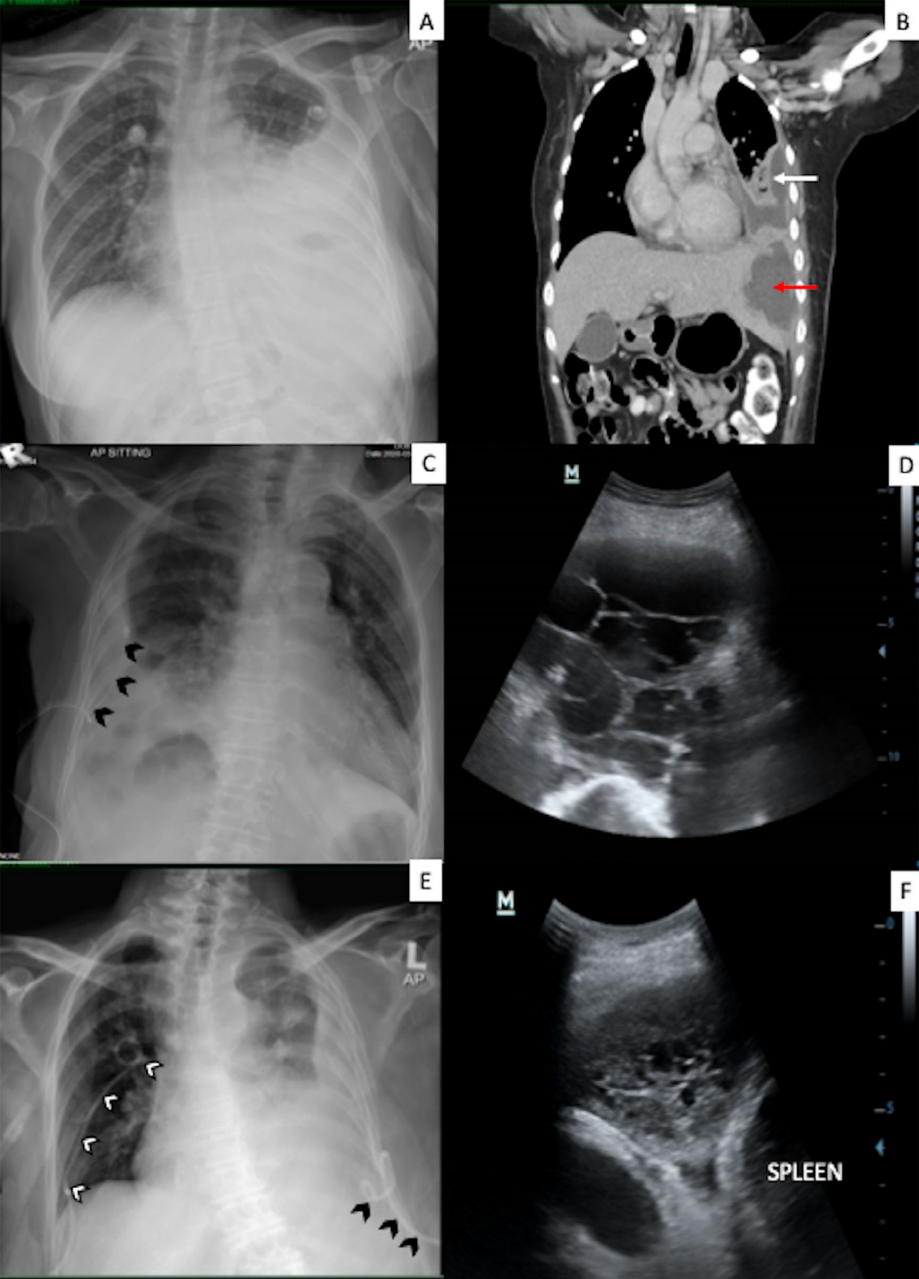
Imaging before intrapleural fibrinolysis. Patient 1: Chest radiograph showed massive left pleural effusion (A) and computed tomography (CT) (B) showed loculated left effusion (white arrow) and hepatomegaly with liver abscess (red arrow). Patient 2: Chest radiograph (C) showed moderate left effusion with chest catheter in situ (black arrows). Ultrasound of patient 2 (D) showed multiseptated effusion. Patient 3: Chest radiograph (E) showed loculated left effusion with left pigtail (black arrows) and right pigtail (white arrows) in situ. Ultrasound (F) showed complex effusion.
